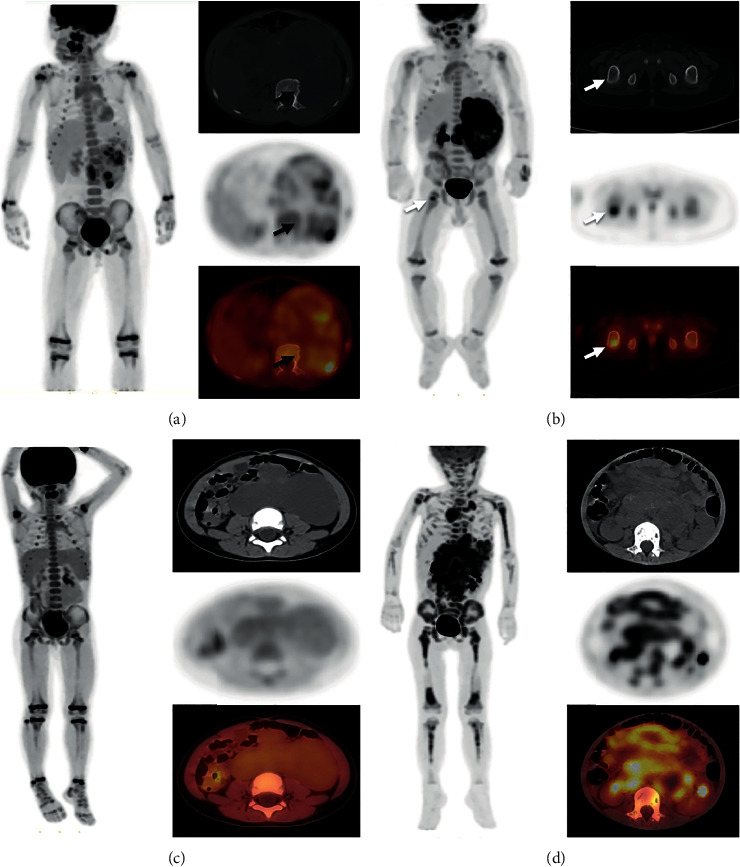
^18^F-FDG PET/CT bone marrow uptake pattern (BMUP). (a) BMUP1 was defined as direct invasion or being surrounded by adjacent tumor tissue (black arrow). (b) BMUP2 was defined as focal/multifocal uptake (white arrow), which was one or more (less than 30) restricted area(s) of FDG uptake. (c) BMUP3 was defined as diffuse and homogeneously increased FDG uptake within the skeleton. (d) BMUP4 was defined as diffuse and heterogeneously increased FDG uptake within the skeleton.
